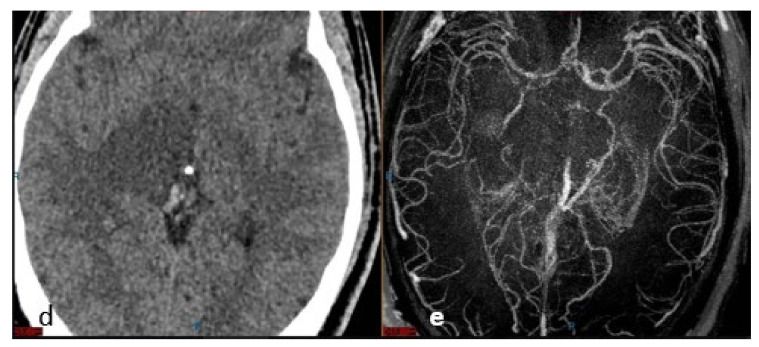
Ischemic lesion of the right thalamus. (a) FLAIR image, axial plane (spontaneous hypersignal of the right thalamus); (b) diffusion-weighted imaging; (hypersignal of the right thalamus); (c) nonenhanced T1 image, sagittal plane; (hyposignal of the right thalamus); (d) nonenhanced CT image (hypodensity of the right thalamus); (e) thrombosis of the right internal cerebral vein. Note the presence of left frontal sinusitis.
